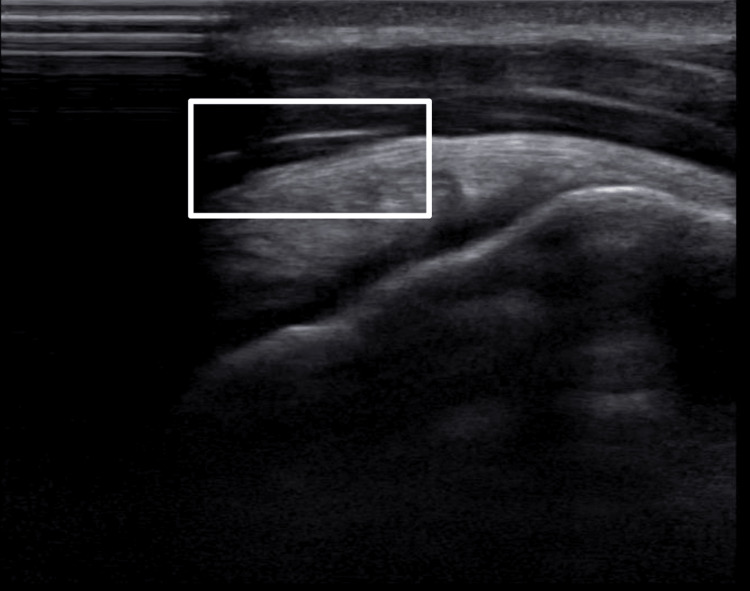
Second post-treatment ultrasound view of the right anterior supraspinatus
